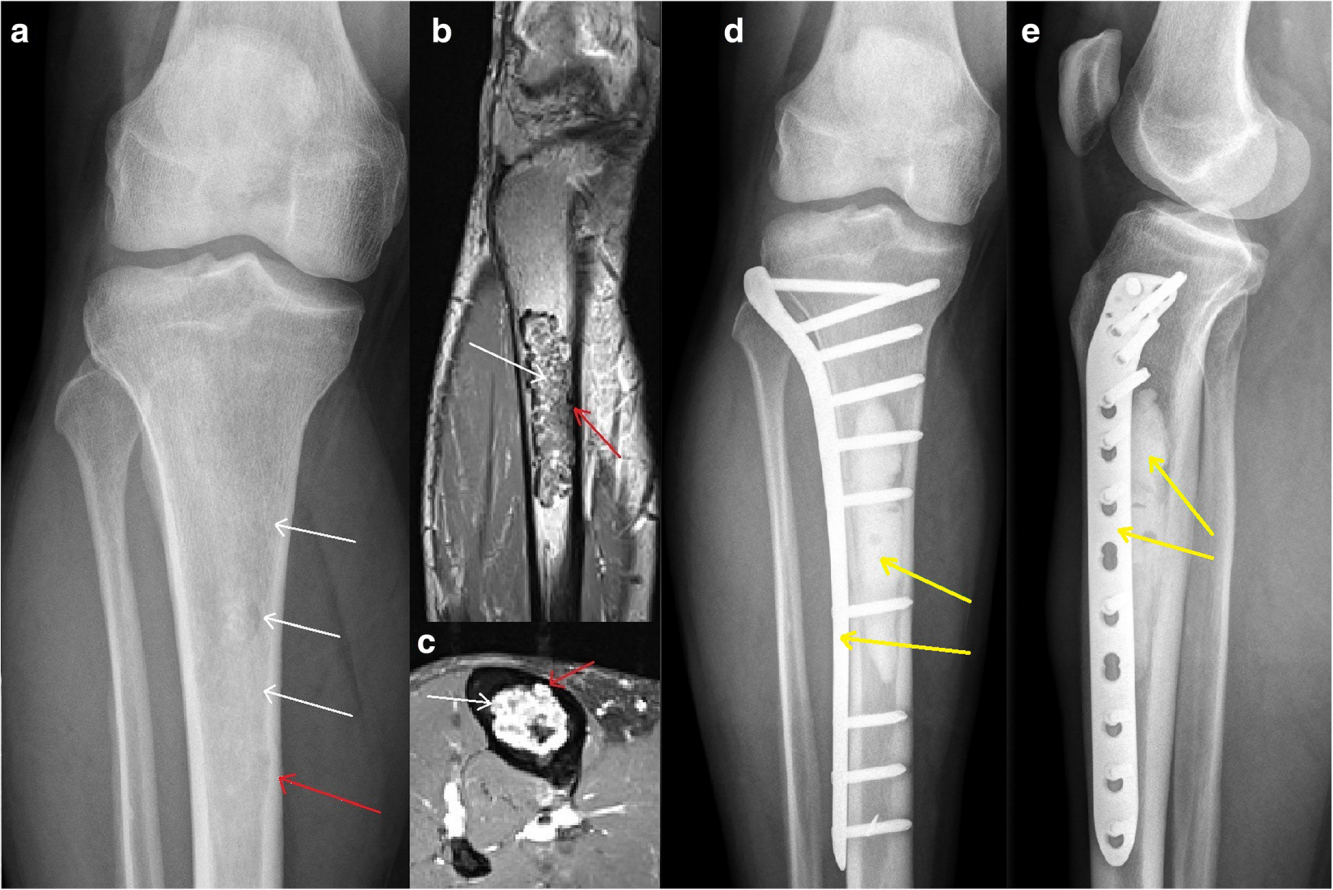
Surgical treatment at the proximal tibia Typical chondroid matrix (white arrows) and endosteal scalloping (red arrows) is visible in ap x-ray (a) and coronal (b) and axial (c) T1 dotarem contrast-enhanced MRI. As a result of the large biomechanical stresses on the lesion location, a compound osteosynthesis using polymethylmethacrylate bone-cement and a plate (yellow arrows in ap (d) and lateral (e) x-rays) was performed after rigorous curettage and use of a high-speed burr.
